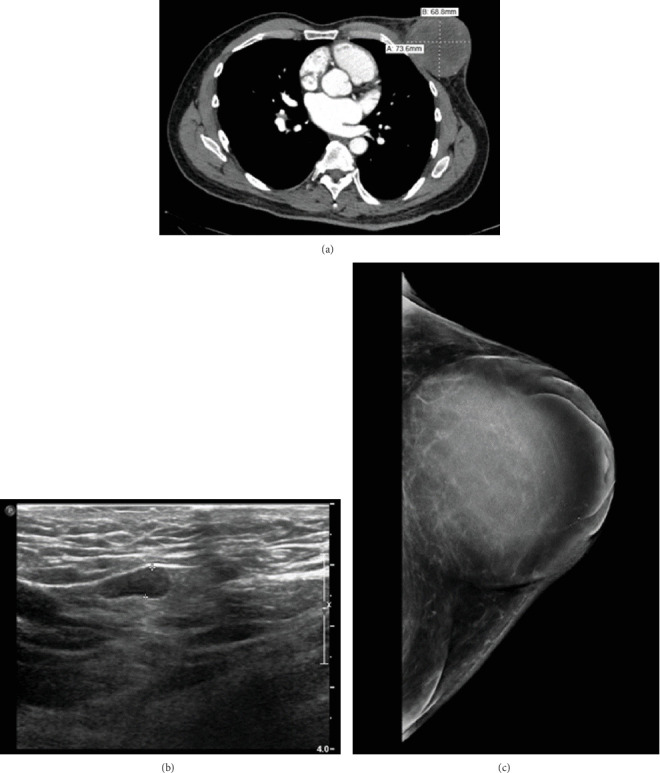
(a) CT shows a soft tissue mass along the left anterior chest wall measuring 7.4 × 6.9 cm. (b) US shows abnormal left axillary lymph nodes with cortical thicknesses measuring 5 mm. (c) Mammogram shows a 7.1 × 6.9-cm solid mass in the left breast centered at 12:00 position 4 cm from the nipple.
